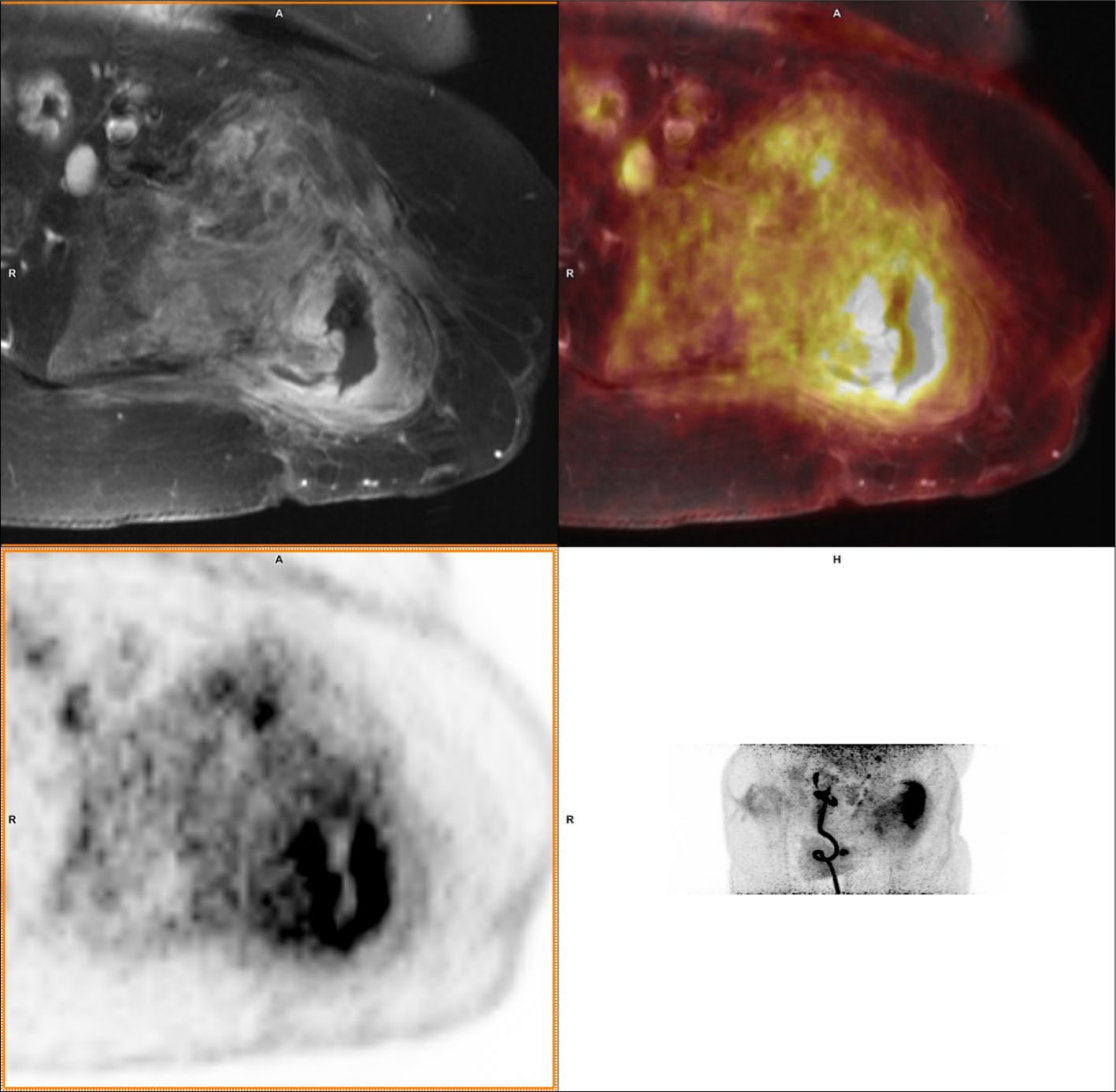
A 43 year old male with a 20 year old complex bone injury. The proximal femur was destructed and soft tissue around the bone showed high FDG uptake. PET and MRI conclusions were consistently positive for osteomyelitis. In this case the extent of the infection based on PET/MRI guides the clinician planning surgery. The presence of osteomyelitis was confirmed by both intraoperative cultures and follow-up
